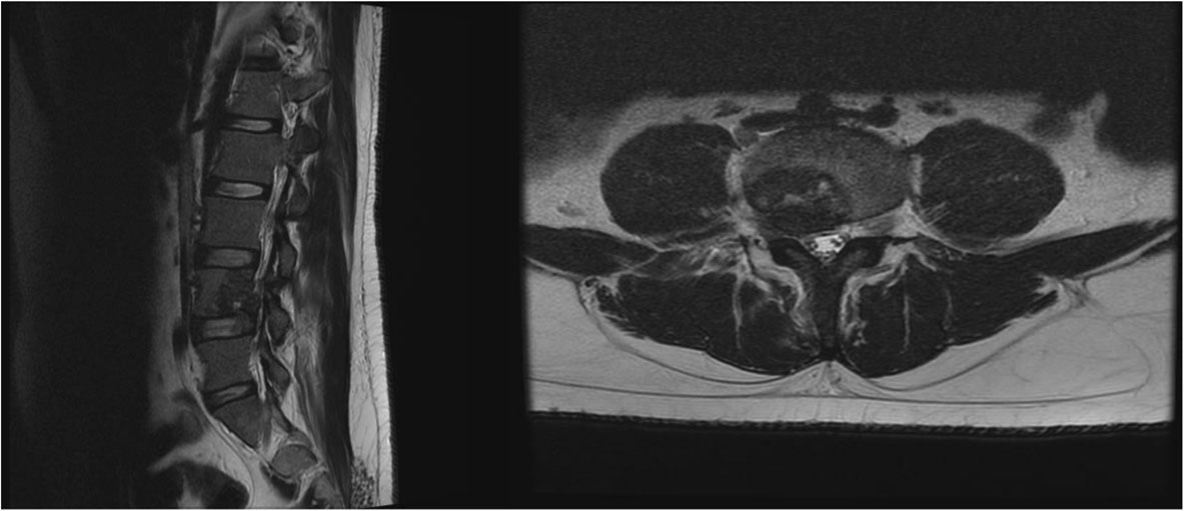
Repeat magnetic resonance imaging at our institution revealed interval increase in size of destructive lesions involving the fourth lumbar vertebral body and fourth lumbar spinous process. Enhancement along the right aspect of the cauda equina extending cephalad from the level of the epidural soft tissue extension at fourth lumbar was also noted
